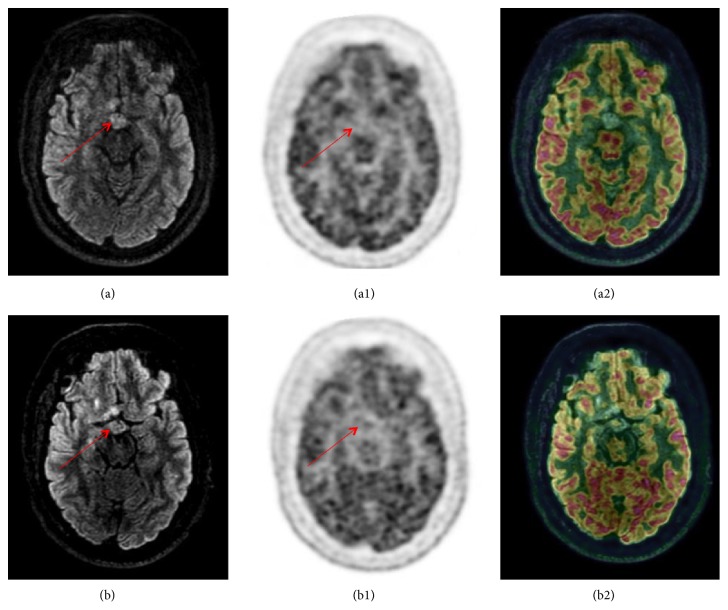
Axial PET-MRI brain imaging.* Axial PET-MRI brain imaging with [18F]FDG at M32 (a, a1, a2) and at M35 (b, b1, b2) of follow-up.
